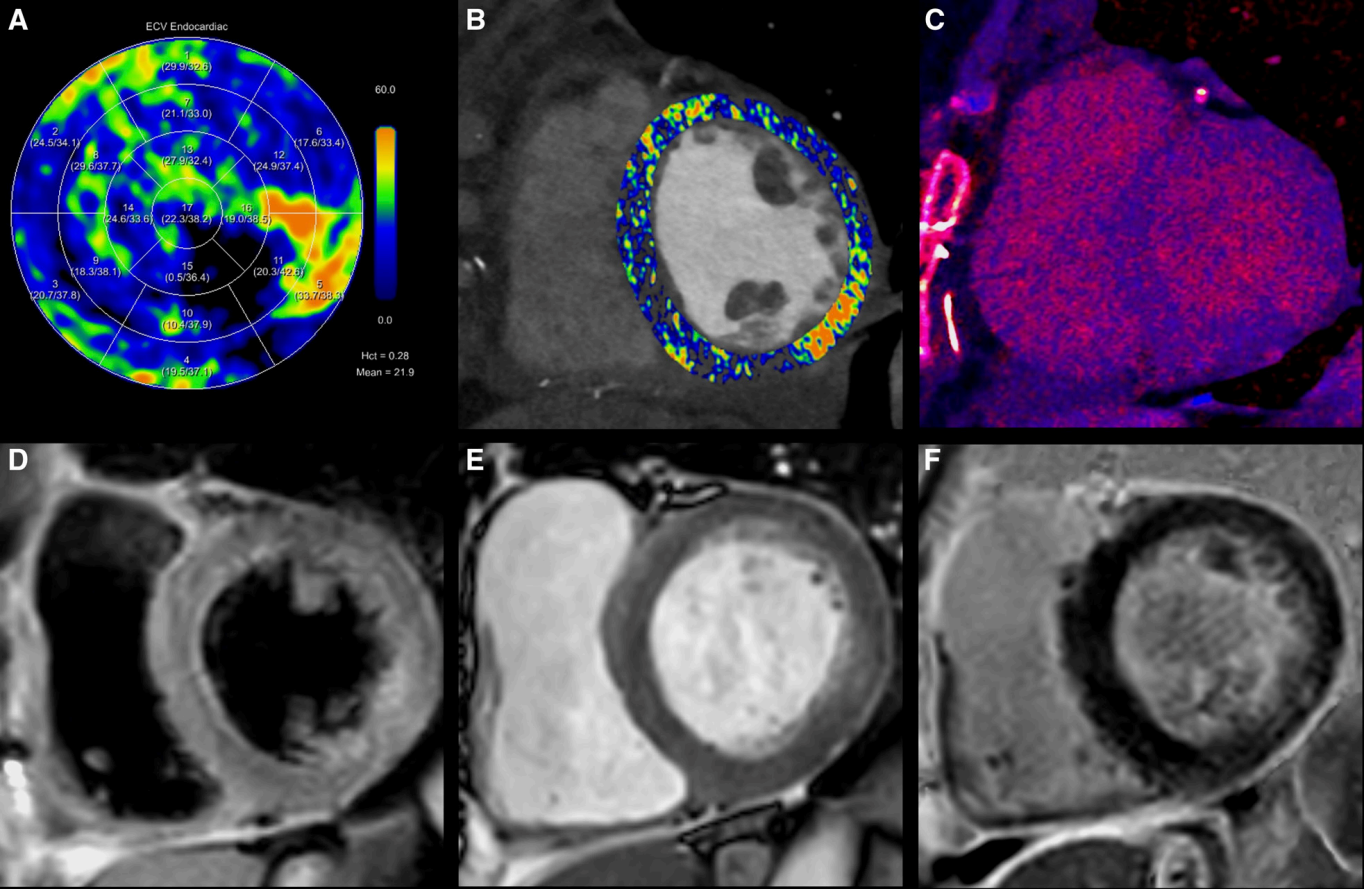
Comparison between photon-counting detector computed tomography (PCD-CT) and cardiovascular magnetic resonance (CMR) imaging findings. PCD-CT extracellular volume (ECV) maps show a focal ECV increase in the basal inferolateral segment (A and B), alongside late iodine enhancement on a calculated iodine map (C). CMR imaging validates these findings by providing the following results in the basal inferolateral segment: increased signal intensity on a T2-weighted sequence with fat suppression suggestive of edema (D), increased signal intensity and wall motion abnormalities on a short axis cine image after gadolinium administration (E), and late gadolinium enhancement (F).
